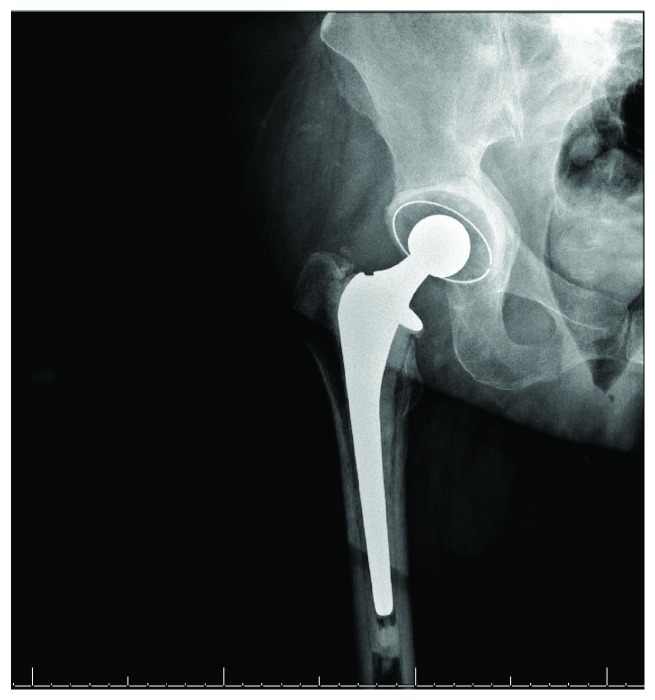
AP radiograph right hip obtained 3 weeks after revision total hip arthroplasty demonstrating no evidence of component loosening.
